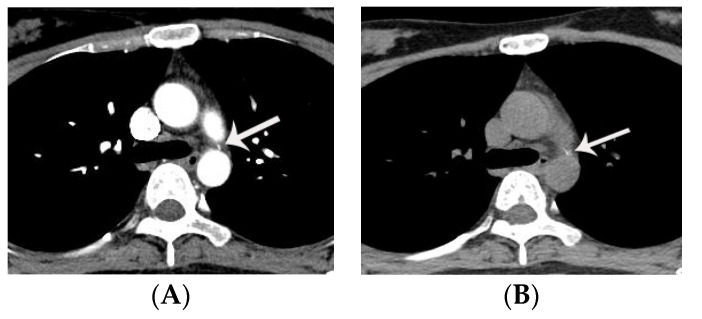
Typical case of calcified ligamentum arteriosum in a 32-year-old female patient. Calcification within the ligamentum arteriosum may be confused with a small PDA if only enhanced chest CT is obtained. Note calcification (arrow on A,B) within the ligamentum arteriosum at the level of tracheal bifurcation.
